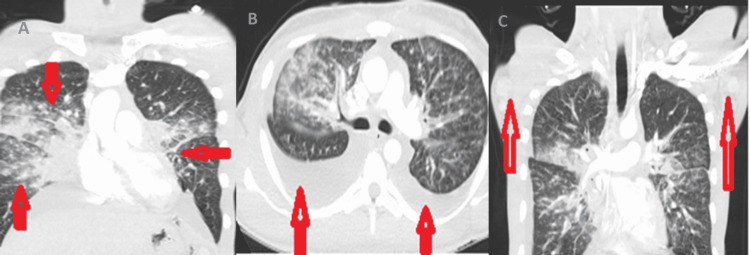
A contrast-enhanced CT chest (A) Coronal image: The arrows show diffuse bilateral airspace opacities concerning atypical multifocal pneumonia. (B) Axial image: The vertical arrows are pointing to bilateral pleural effusions. (C) Coronal image: Vertical arrows point toward the necrotic-looking enlarged axillary lymph nodes that were suspicious of a disseminated infectious or neoplastic process.
